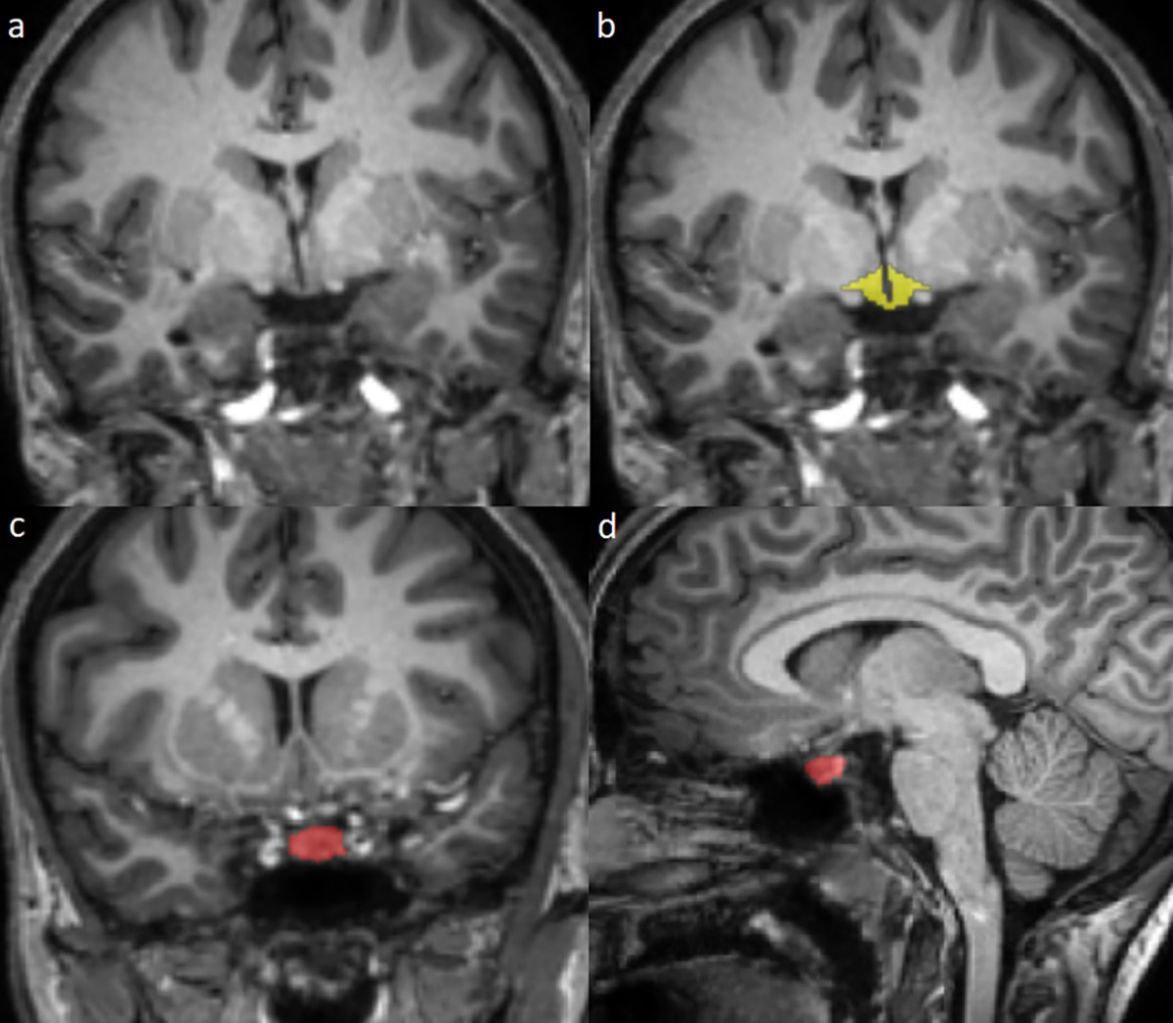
Noncontrast T1-weighted MR images of the hypothalamus and pituitary gland. The hypothalamus is shown without (a) and with superimposed segmentation in yellow (b). Segmentation of the pituitary gland (red) is shown in the coronal (c) and sagittal (d) planes.
